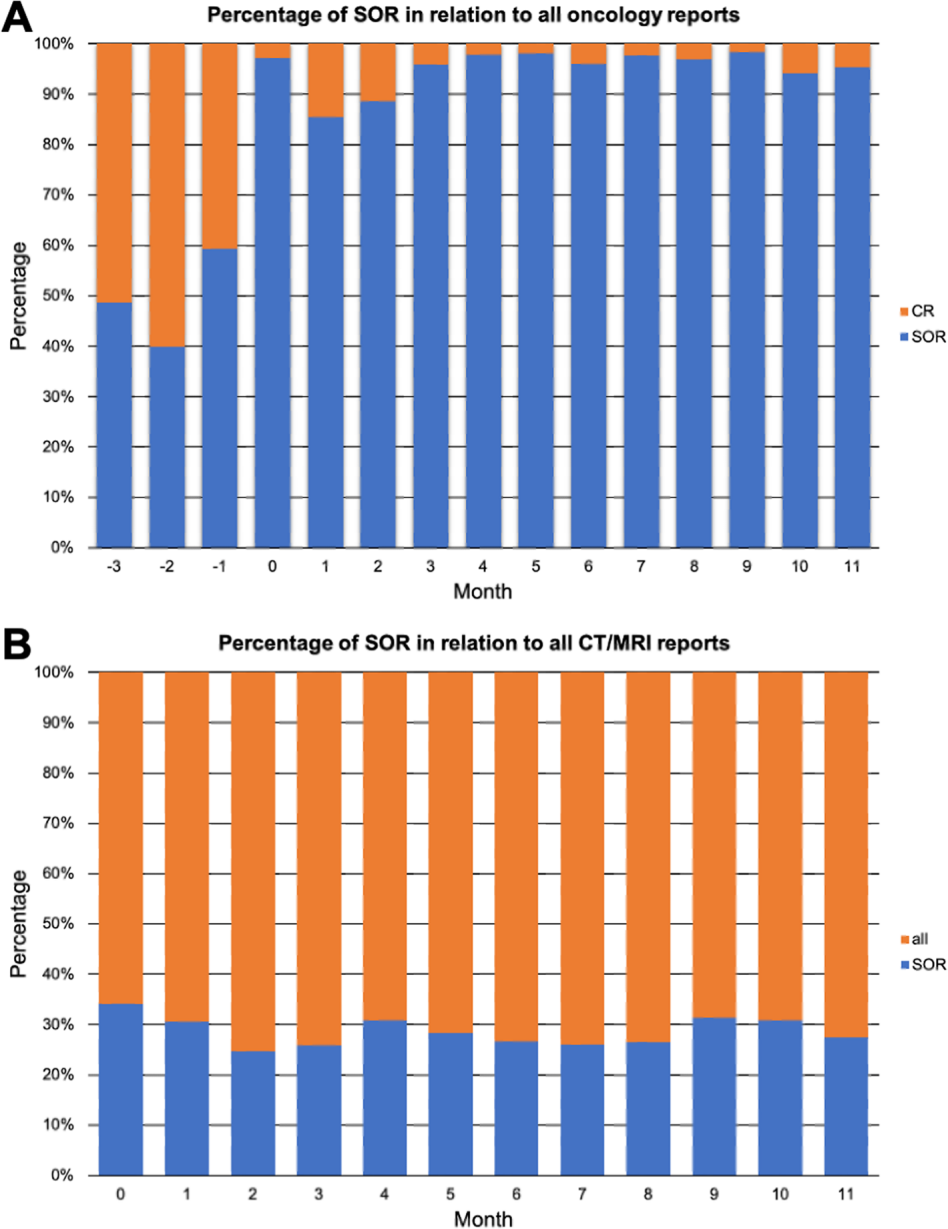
Percentage of structured oncology reports used for oncological follow-up imaging. a The proportion of SOR to all reports generated for a representative division of the department for medical oncology. b The proportion of SOR to all reports generated for scans at the CT and MRI scanners used for body imaging. Month 0 indicates time point of department-wide roll out of structured oncology reports. SOR, structured oncology report; CR, conventional report
